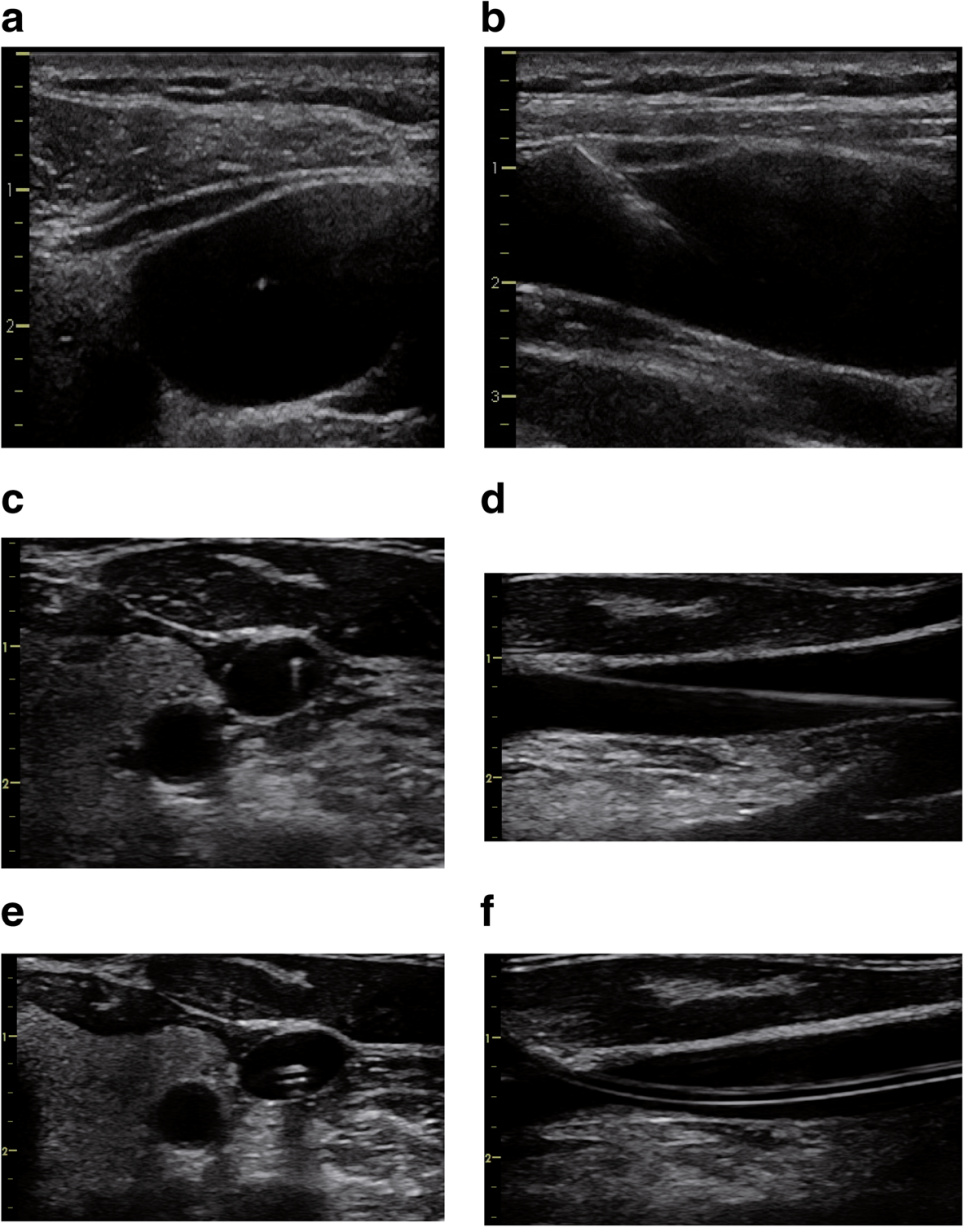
Ultrasound to confirm needle, wire, and catheter position in the vein. Ultrasound images during real-time ultrasound-guided central venous catheter placement in the right internal jugular vein. Ultrasound guidance should include confirmation of the needle position in the vein before approaching the guide wire (short-axis/out-of-plane view (a) and long-axis/in-plane view (b)). In addition, the correct position of the guide wire in the vein (short-axis (c) and long-axis (d)) and the correct position of the catheter in the vein (short-axis (e) and long-axis (f)) should be confirmed
